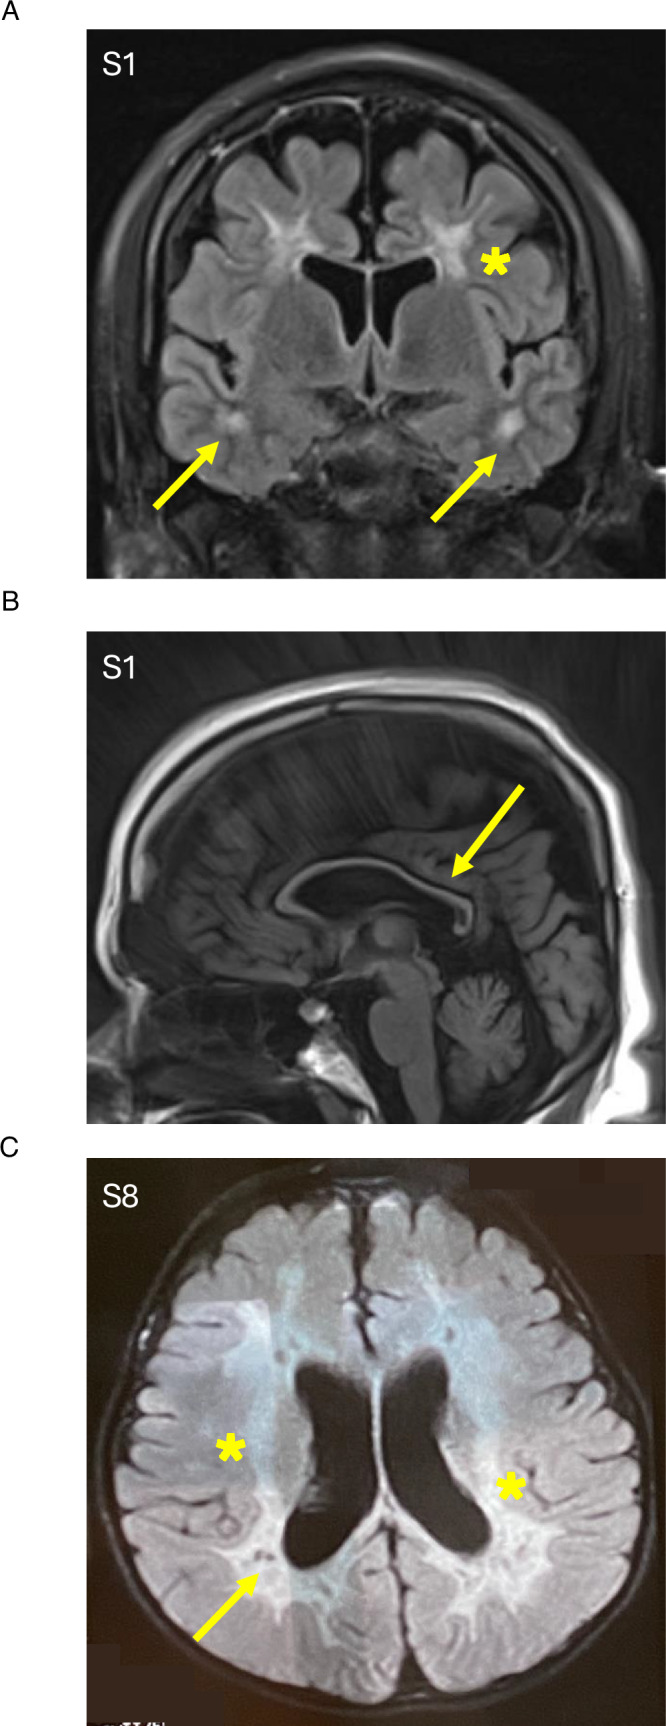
Representative brain MRI findings in *COXFA4* affected subjects. A, B Subject S1 (Family 1), age 25 years. A Coronal FLAIR sequence demonstrating marked periventricular white matter hyperintensities (asterisk) extending into the temporal white matter (arrows). B Sagittal view highlighting corpus callosum atrophy. C Subject S8 (Family 8), age 2 years. Axial FLAIR sequence showing bilateral, symmetrical hyperintensities in periventricular white matter (asterisk). A few small periventricular cystic changes were also visible (arrows).
